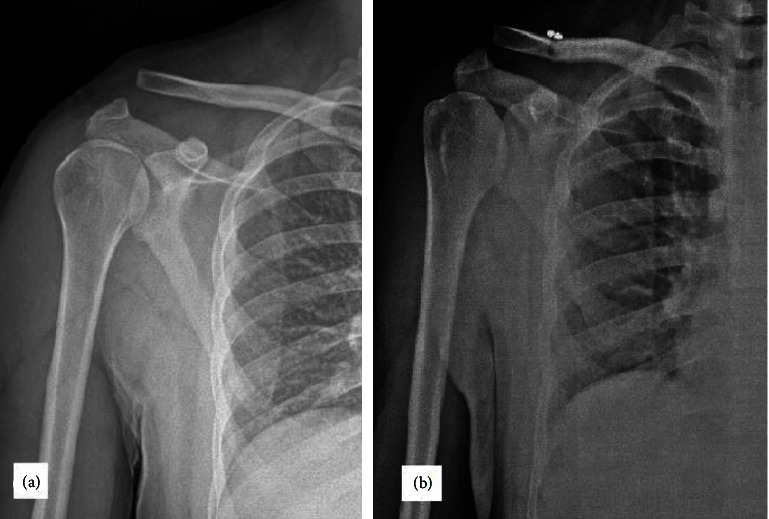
Serial radiographs of a 36-year-old man treated with a minimally invasive TightRope system. (a) Preoperative anterior-posterior radiograph showing type III AC dislocation. (b) 1-month postoperative follow-up with reduction failure.
